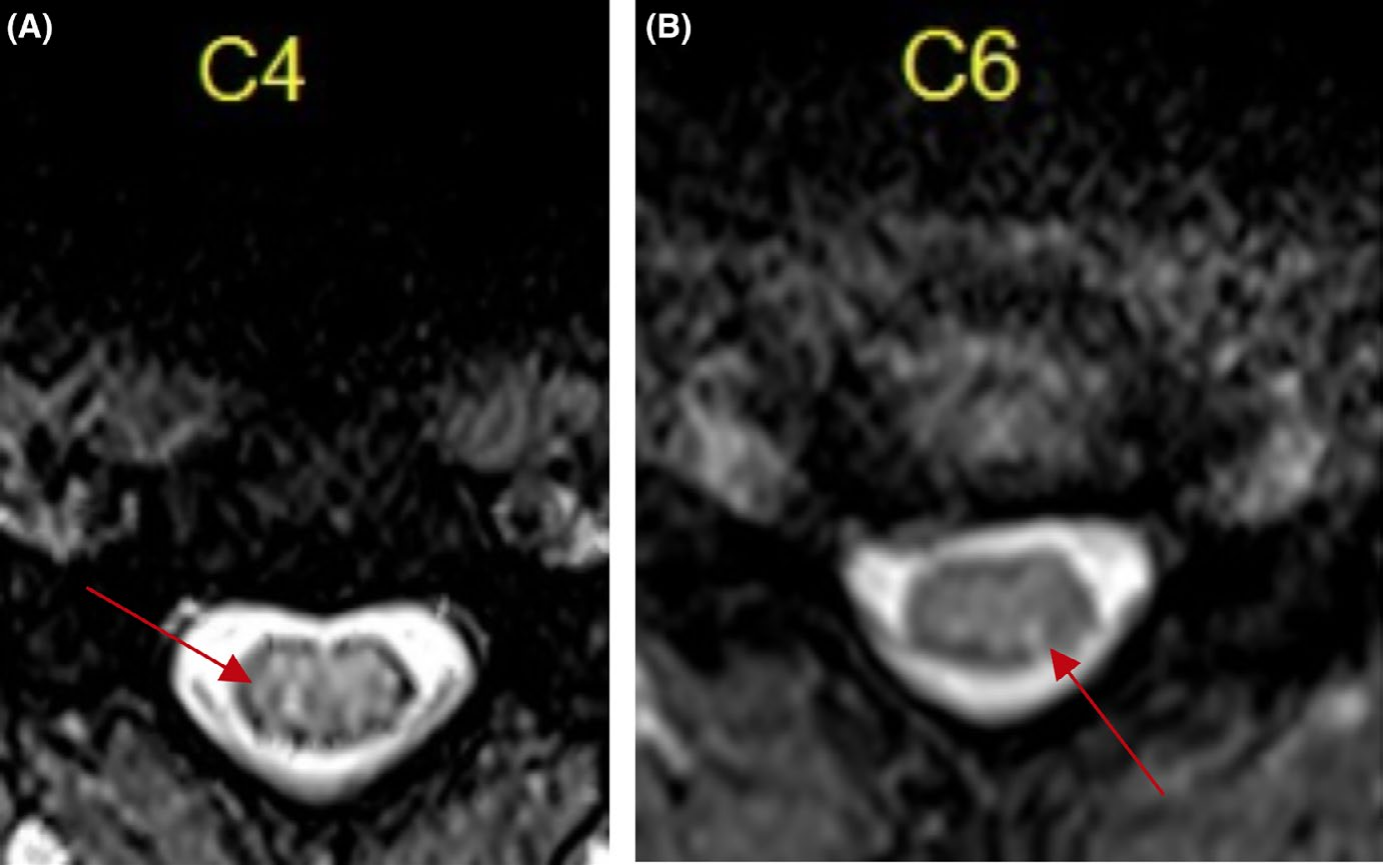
Axial T2 MRI gradient axial shows diffuse abnormality at (A) C4 and (B) C6 levels
